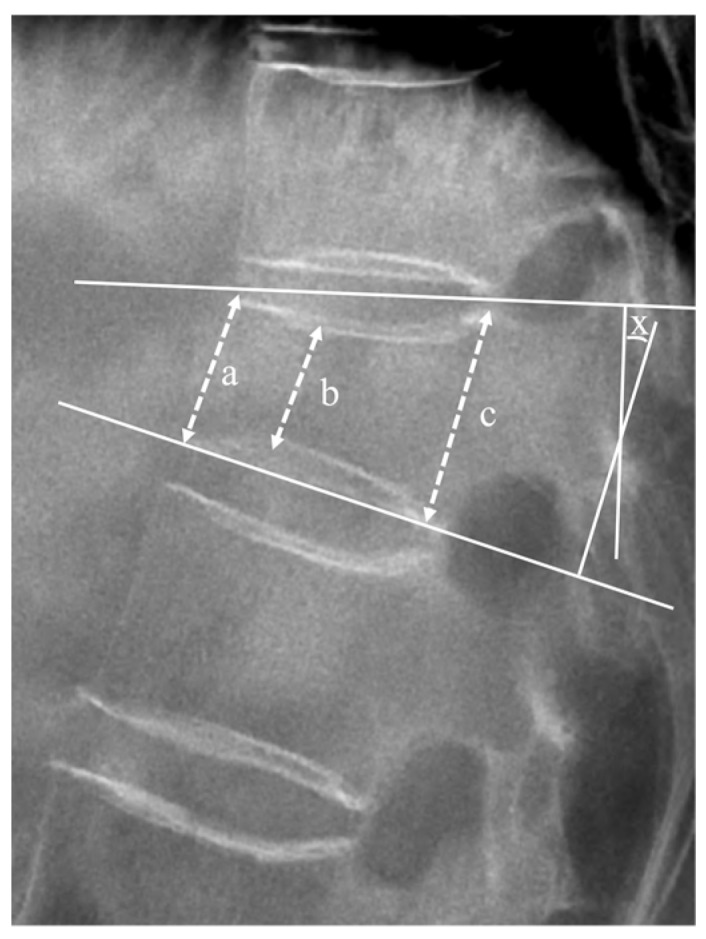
Lateral plain radiograph displaying measured factors. The mean height of the vertebral body was defined as the average of anterior margin (a), minimal height (b), and posterior margin (c) of the vertebral body, expressed as (a + b + c)/3. The vertebral height ratio (%) was calculated using the following equation: ($\frac{mean\;height\;of\;the\;index\;level}{\frac{mean\;height\;of\;the\;upper\;adjacent\;body\;+\;mean\;height\;of\;the\;lower\;adjacent\;body}{2}}$) × 100. The segmental angle (X) was measured as the angle formed by lines drawn along the superior and inferior plateaus of the vertebral body, where they intersect.
